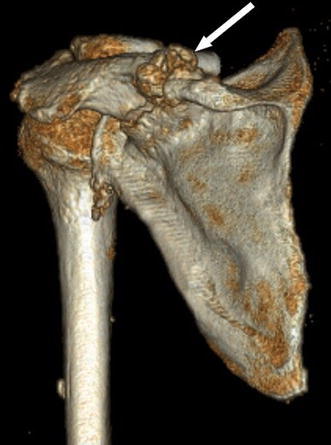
(Patient 1) CT reconstruction of left shoulder showing scapular spine fracture (*white arrow*)
